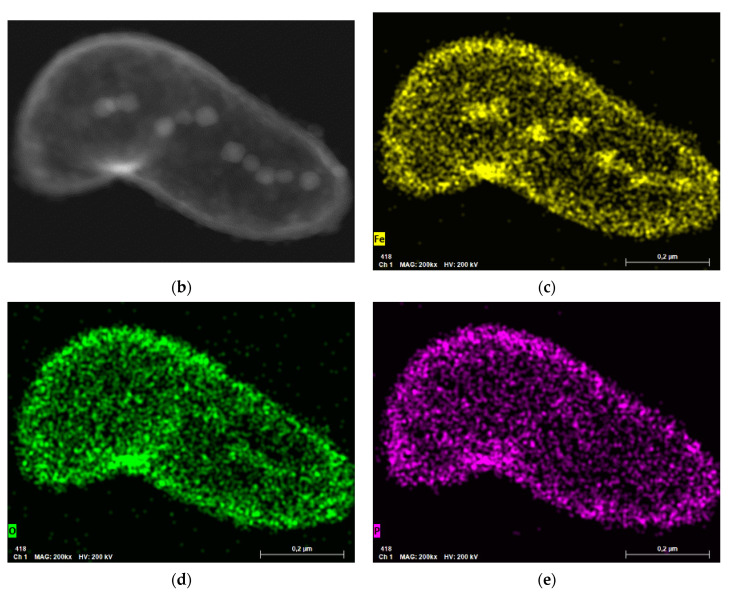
Analysis of the elemental composition of magnetotactic bacteria (MTB) lyophilizate: (a) X-ray energy dispersive analysis results; (b) internal structure of MTB cell fragment; (c–e) X-ray Fe, O, and P mapping of an MTB cell, respectively.
